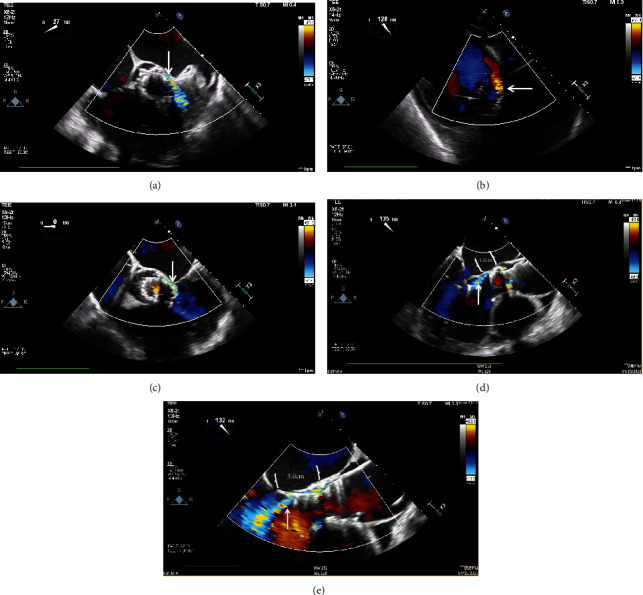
Transesophageal echocardiogram after valve repositioning. Mild PVL by color Doppler (arrow) in the (a) short-axis and (b) deep-transgastric views (Videos 4 and 5). (c) PVL circumferential extent (arrow) was reduced to 20%. (d) Long-axis view showing mild PVL (arrow). (e) Severe PVL (arrow) prior to valve repositioning for comparison. Postrepositioning, the aortic annulus-to-valve inflow distance (braces) was reduced from (e) 3.0 cm to (d) 1.3 cm.
